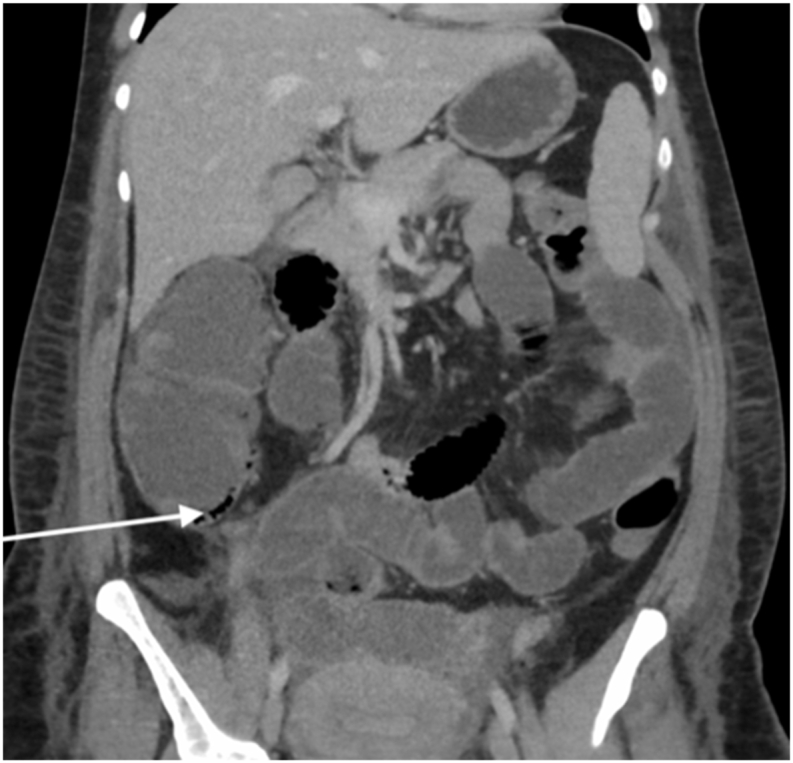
Coronal CT image, the largest diameter of the caecum is 10 cm, intestinal pneumatosis is indicated by 1 arrow.
